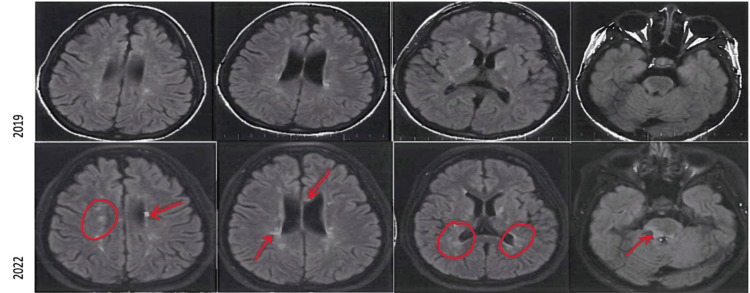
MRI FLAIR sequences from 2019 (above) and 2022 (below) showing increased white matter disease (indicated by red arrows and circles) at periventricular white matter areas, corpus callosum, and brainstem. FLAIR: Fluid-attenuated inversion recovery
